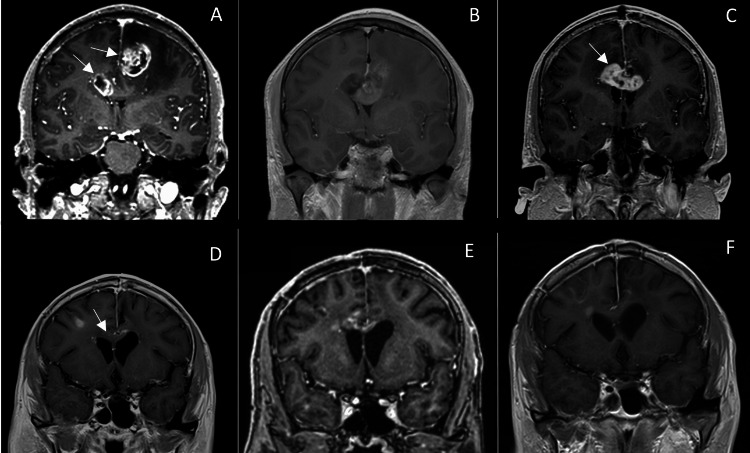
Follow-up MRIs of patient no. 1. (A) Preoperative image – bilateral enhancement suggesting bGG (arrows); (B) Day 1 postoperative image; (C) MRI at three months postoperatively with a contrast-enhanced lesion on the corpus callosum (arrows); (D) MRI at 18 months postop with excellent response and evolution due to oncological treatment with no enhancement on the corpus callosum (arrows); (E) MRI at 24 months after resection surgery; and (F) MRI at 27 months after resection surgery. bGG: Butterfly grade 4 glioma.
